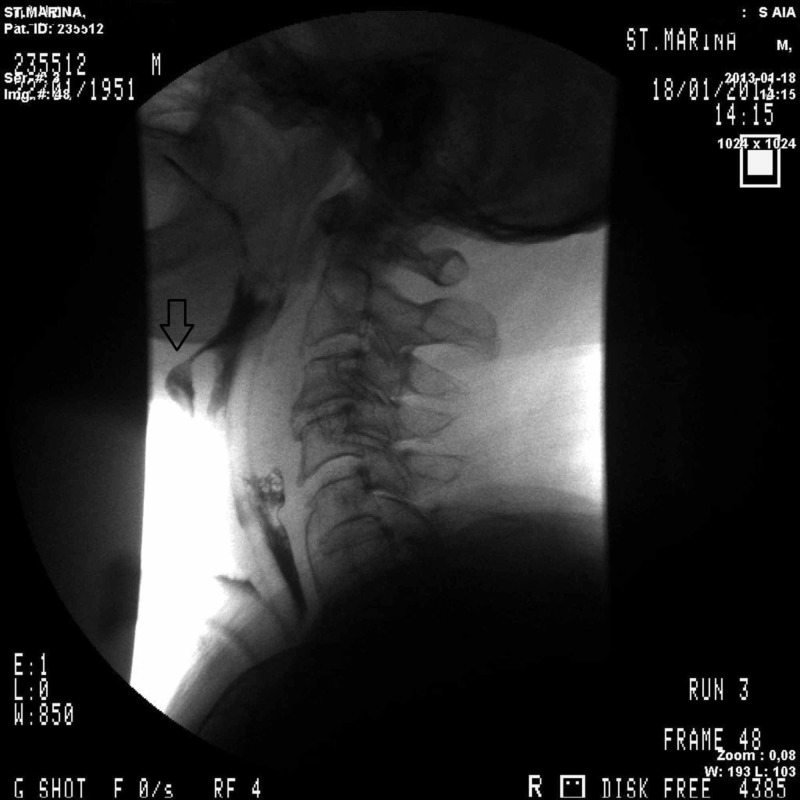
Barium swallow radiographs On sagittal view fistula between the neopharynx and the skin at the C2-C4 level is visualized. A nasogastric tube is in place.
